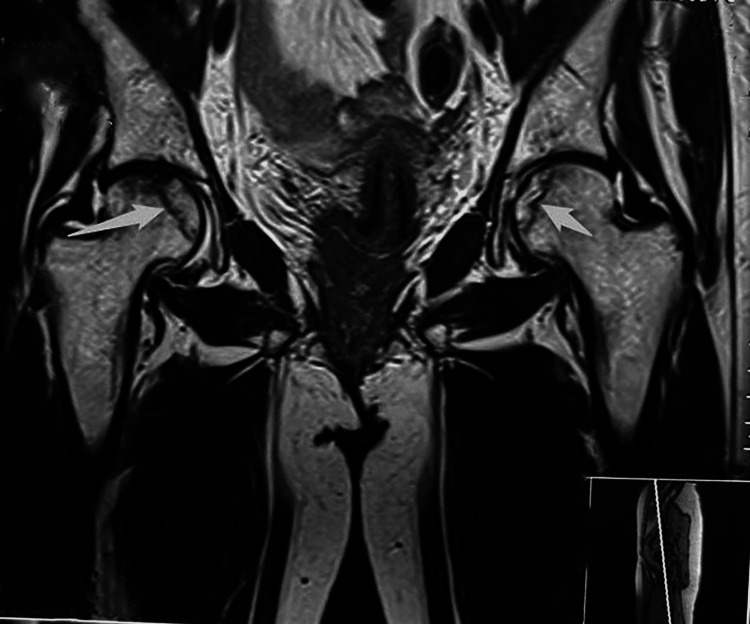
MRI of the pelvis showed bilateral femur head avascular necrosis (white arrow)
